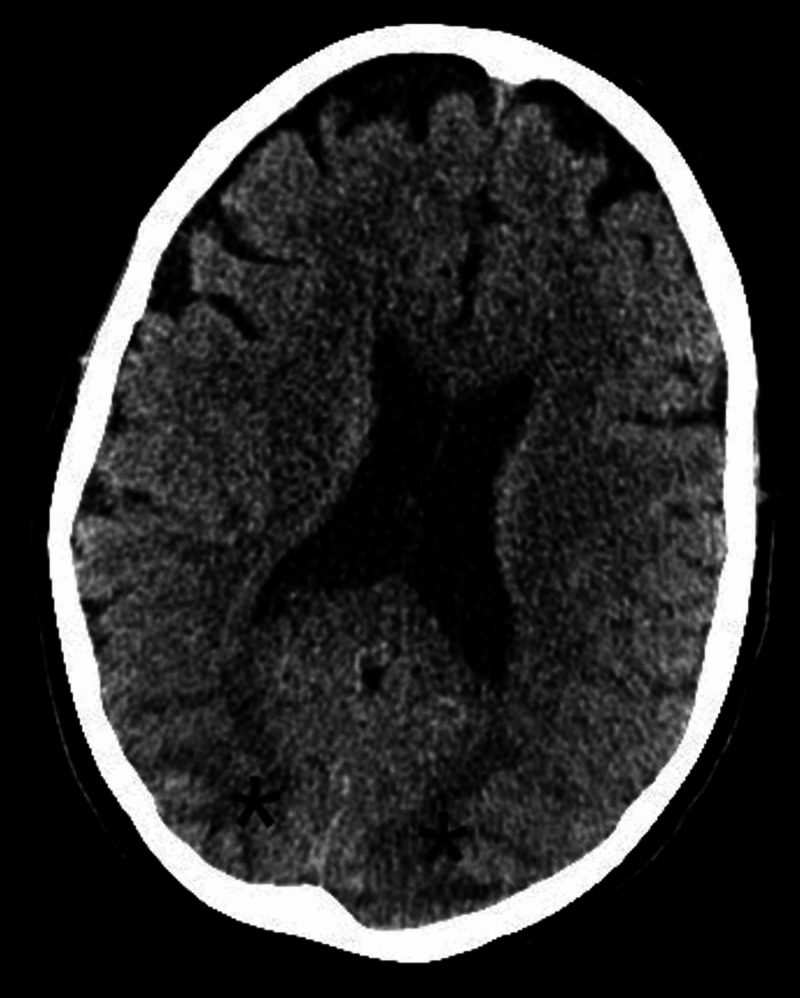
CT scan of the head showing evidence of relative hypodensities (marked with asterisks) suggestive of posterior reversible encephalopathy syndrome (PRES)
